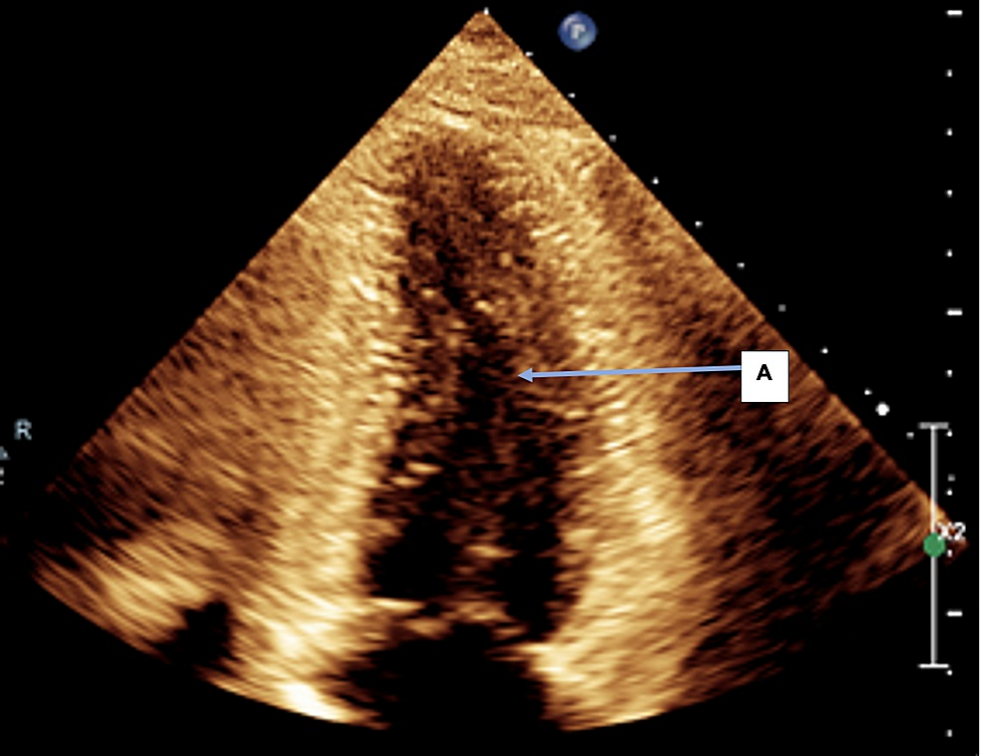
Post-treatment TTE: Apical 4 chamber (A4C) view in systole showing preserved LV systolic function. A: The left ventricle appears to be contracting well in this still image.
